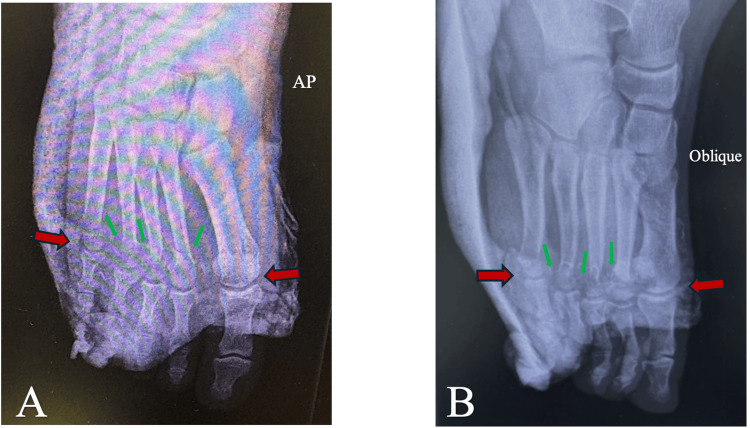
AP (A) and oblique (B) right foot radiographs after reduction of the first and fifth MTP joints (red arrows) and reduction of the neck fractures of the second, third, and fourth metatarsals (green arrows) MTP, metatarsophalangeal
